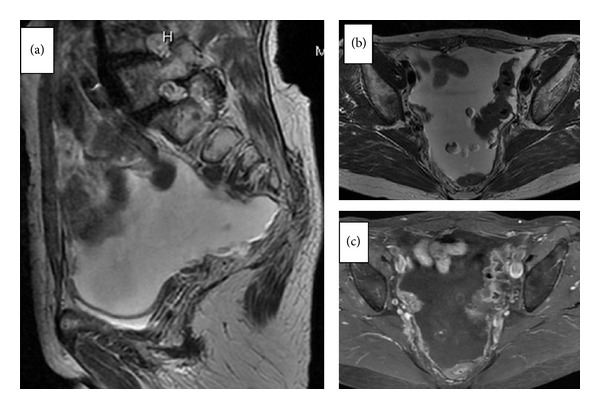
Magnetic resonance imaging (MRI) shows massive ascites and nodular/irregular thickening of the mesentery and peritoneum and a small mass suspected in the right normal ovary and marked enhancement of the papillary projections. (a) T2-weighted images (sagittal view), (b) T2-weighted images (axial view), and (c) Gadolinium-enhanced fat-suppressed turbo spin-echo T1-weighted MR image (axial view).
